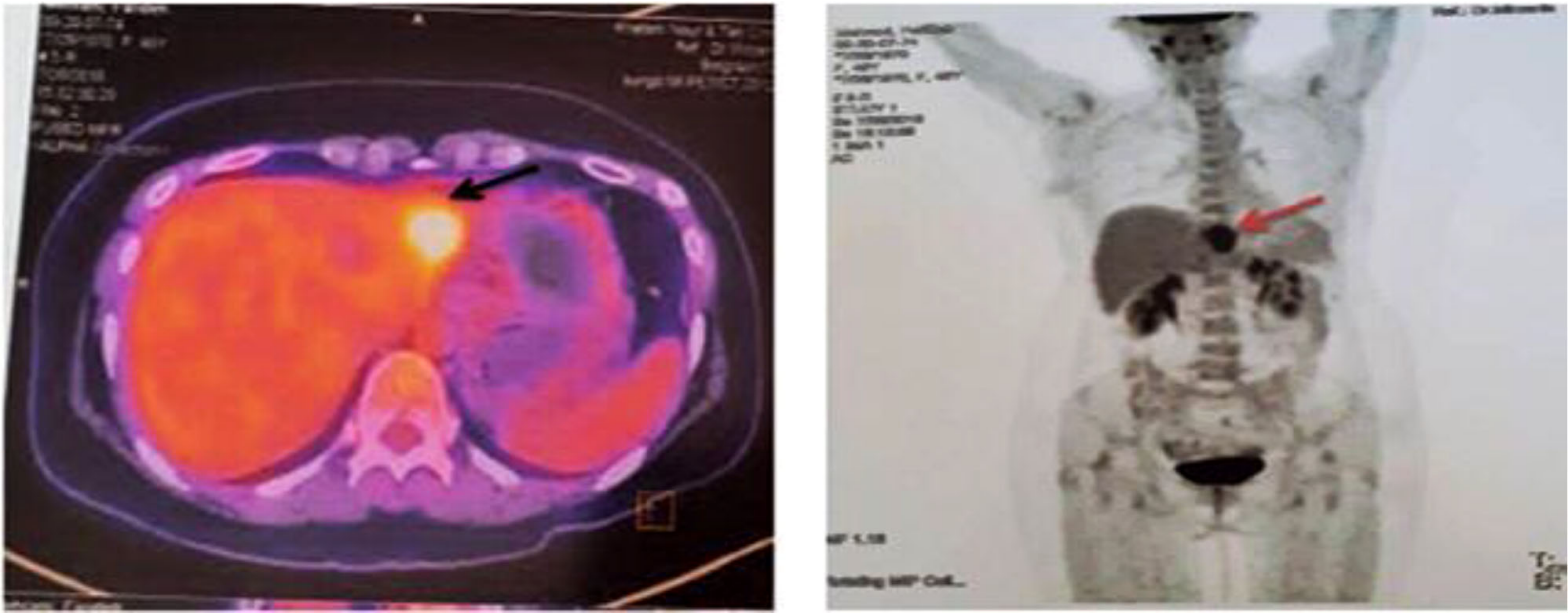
First PET/CT on 26 July 2018. The arrows show a fluorodeoxyglucose (FDG) avidity-standardized uptake value (SUVmax = 9.2) hypo-attenuating lesion with partial necrosis with the left liver lobe, compatible with metastatic disease.
